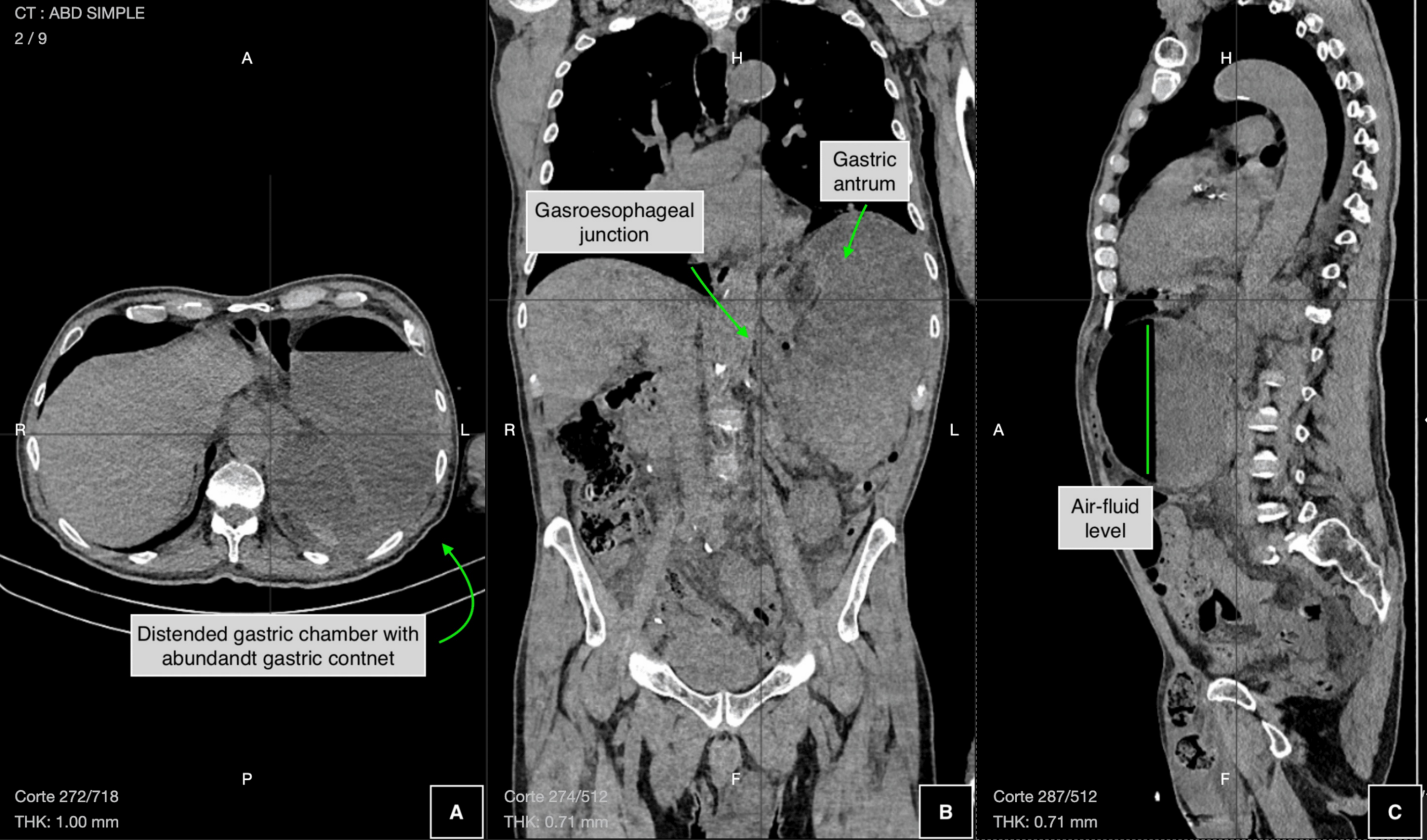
Abdominal CT scan showing mesenteroaxial gastric volvulus (A) Distended gastric chamber. (B) Displacement of the antrum above the gastroesophageal junction. (C) Air-fluid level secondary to gastric obstruction caused by mesenteroaxial volvulus.
